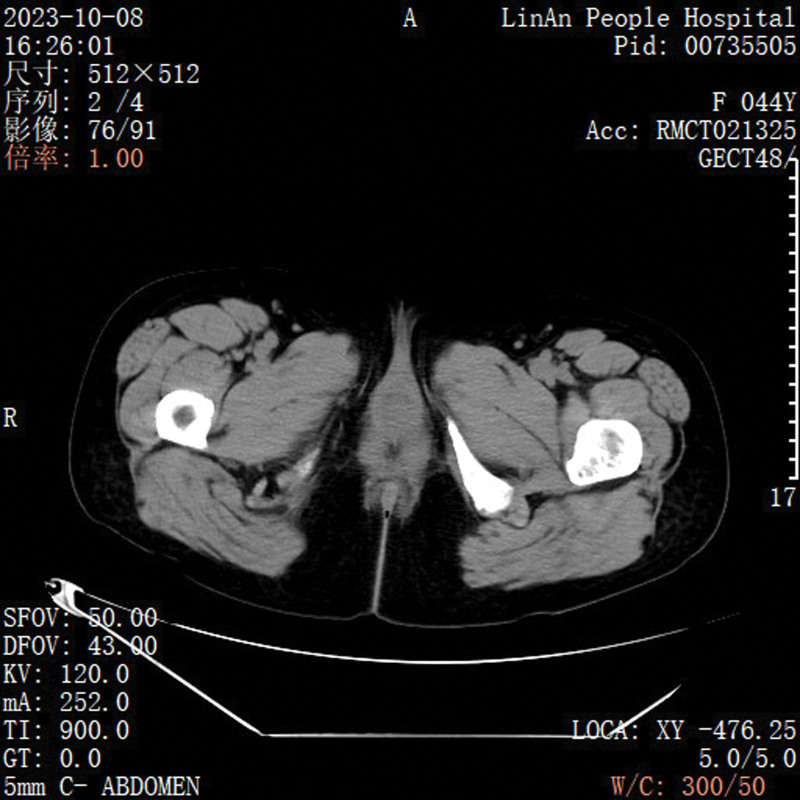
CT scan revealed that the tumor originates from the urethra. CT = computed tomography.
